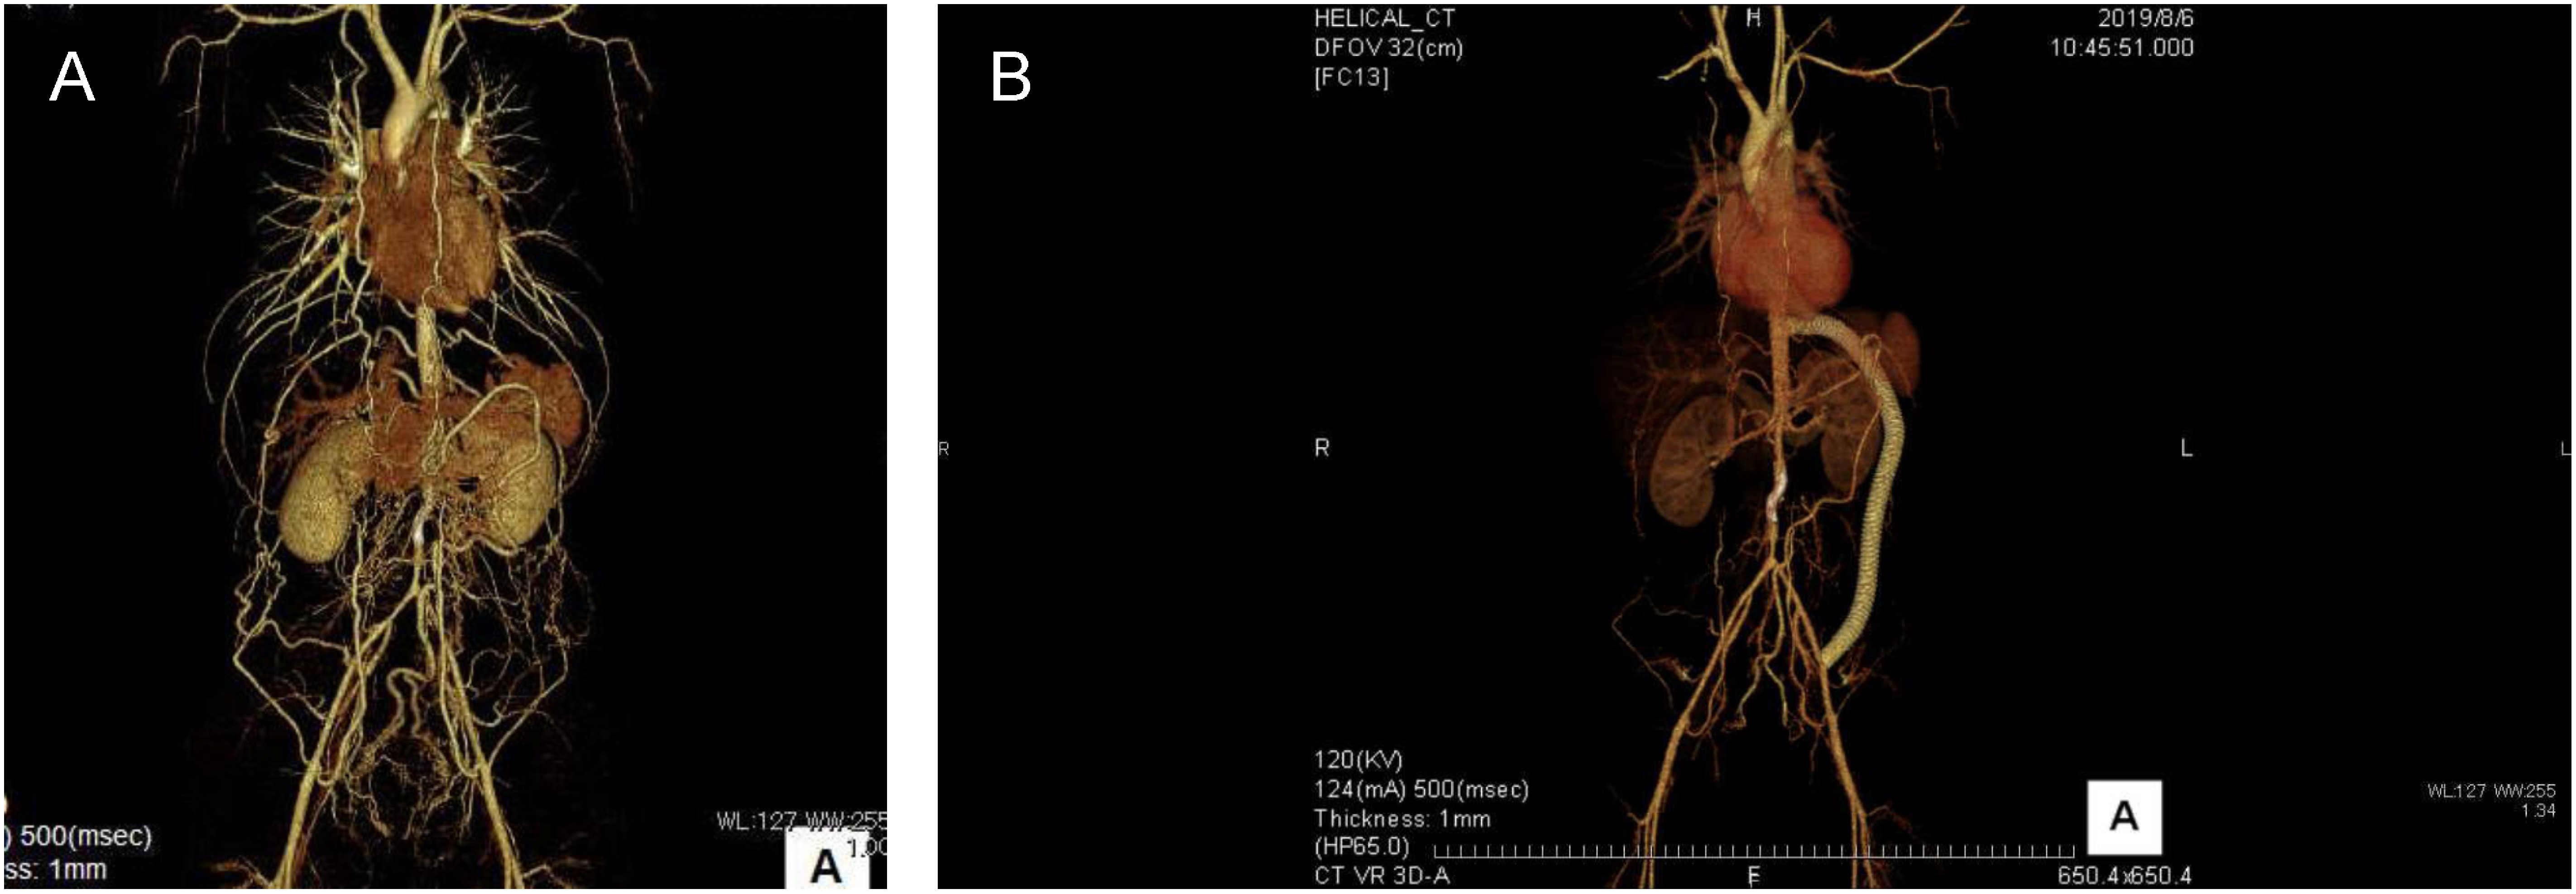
Fig. 3 (A) Enhanced computed tomography before the second surgery shows that both the proximal and distal sites of anastomosis are still too narrow, and there are numerous collateral vessels developing. (B) Enhanced computed tomography following the second surgery 1 year later. Anterior view shows that both grafts are patent and that numerous collateral vessels have developed.
